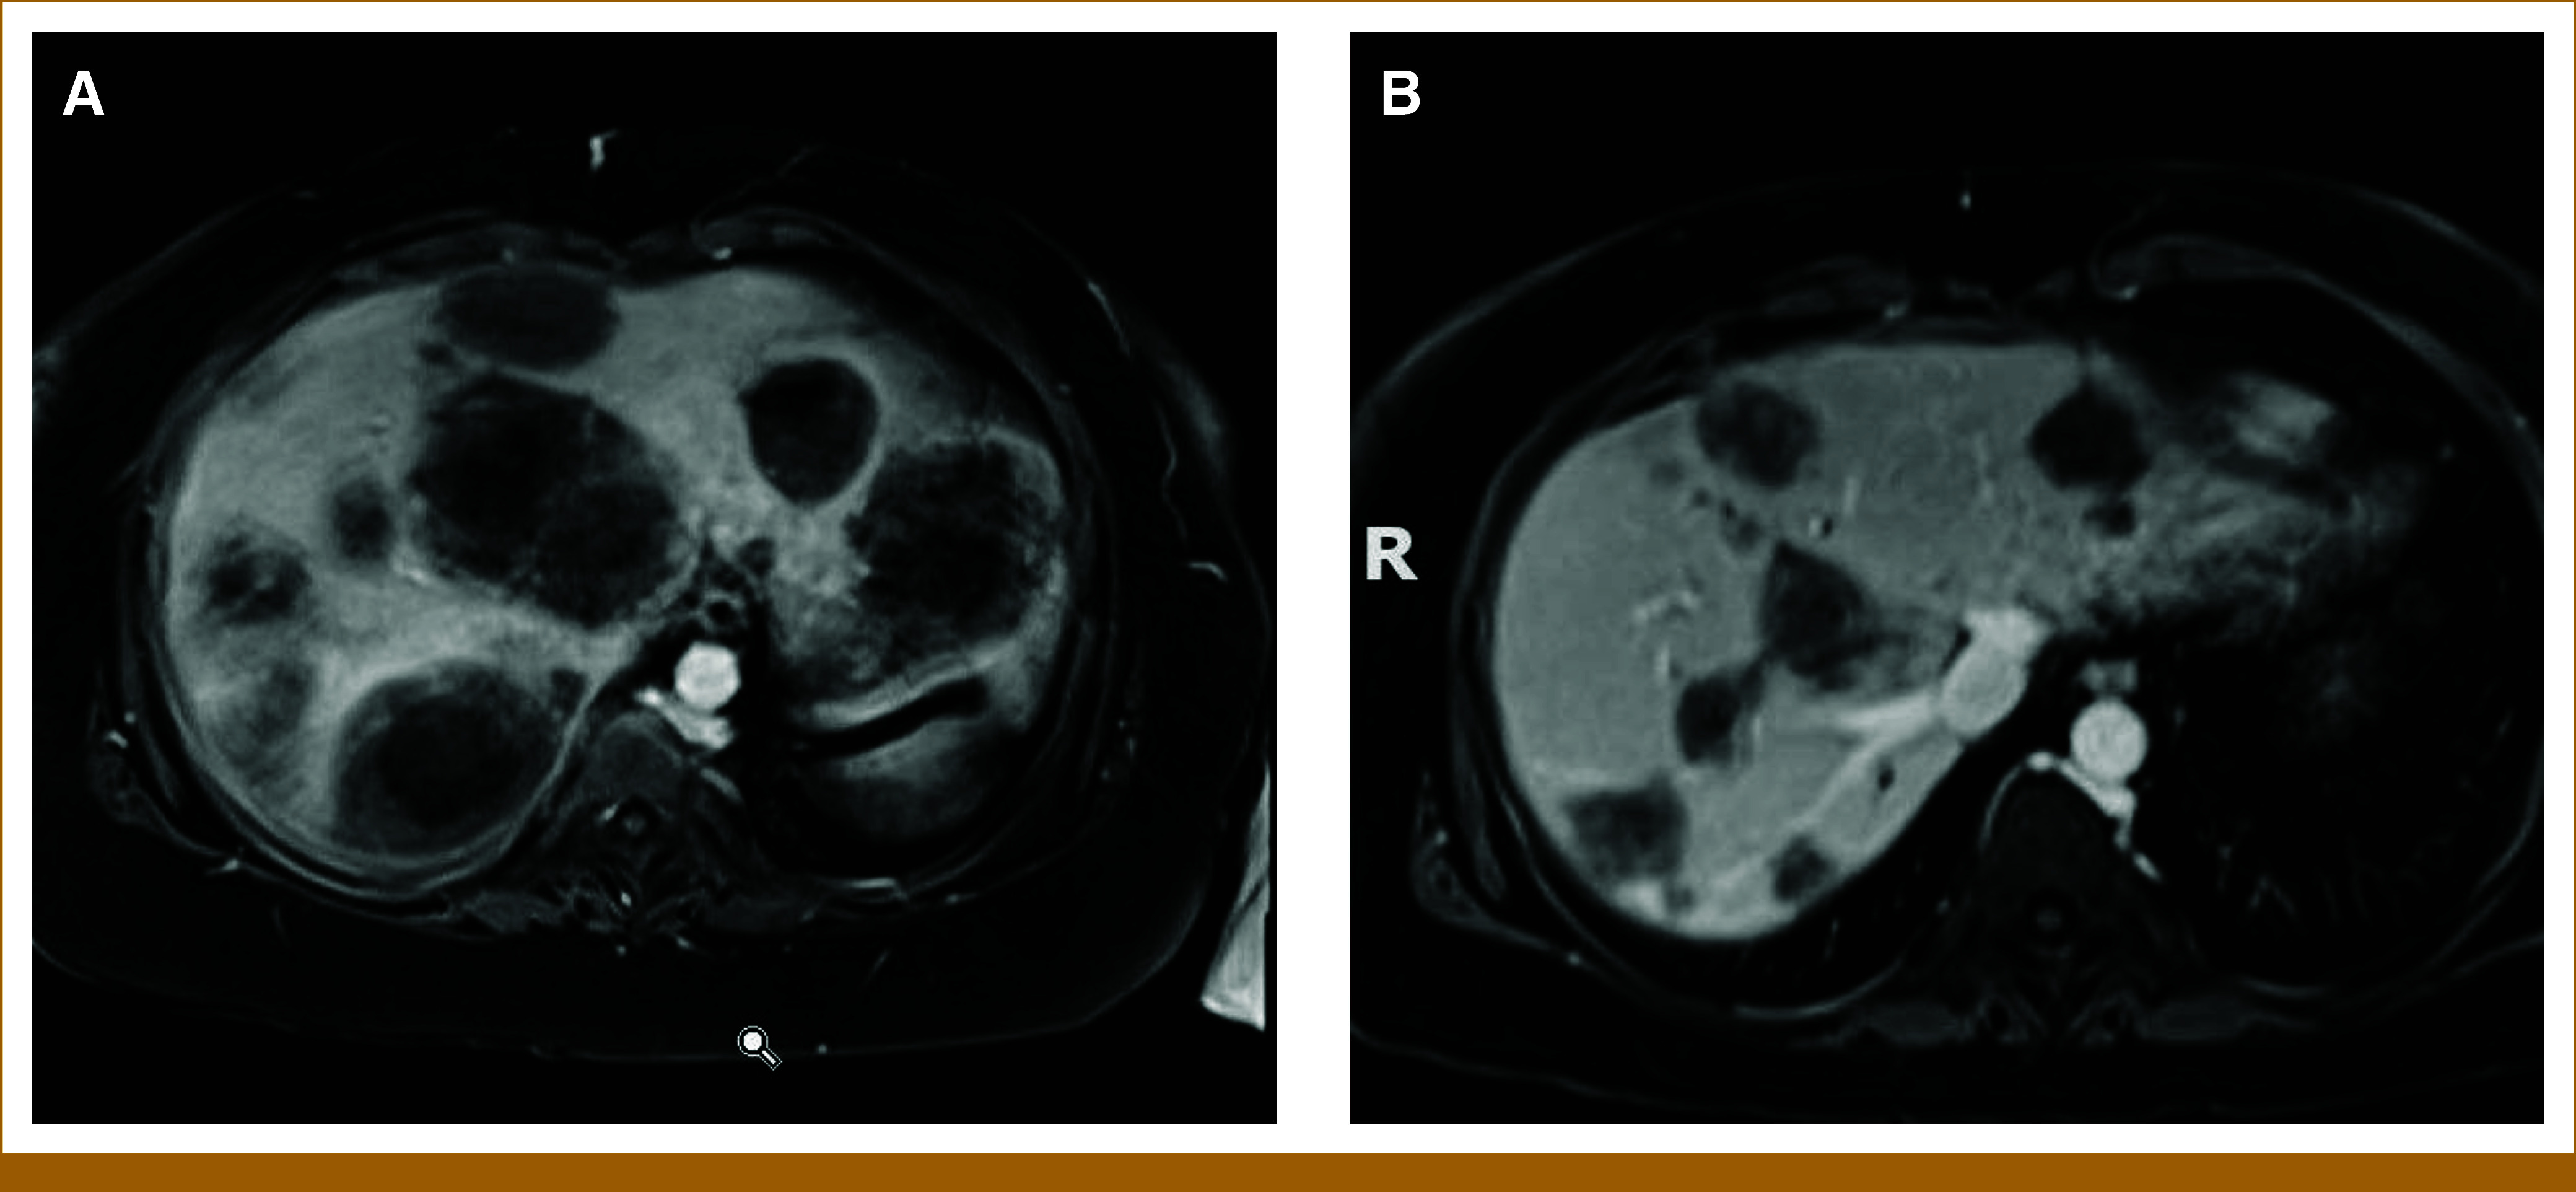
CT imaging showing treatment response to erlotinib in patient 13. Axial CT images (A) at baseline and (B) 2 months post erlotinib in an *ERRFI1*-mutant patient, demonstrating significant reduction in hepatic metastases. This represents a partial response in a heavily pretreated patient with refractory biliary tract cancer.
